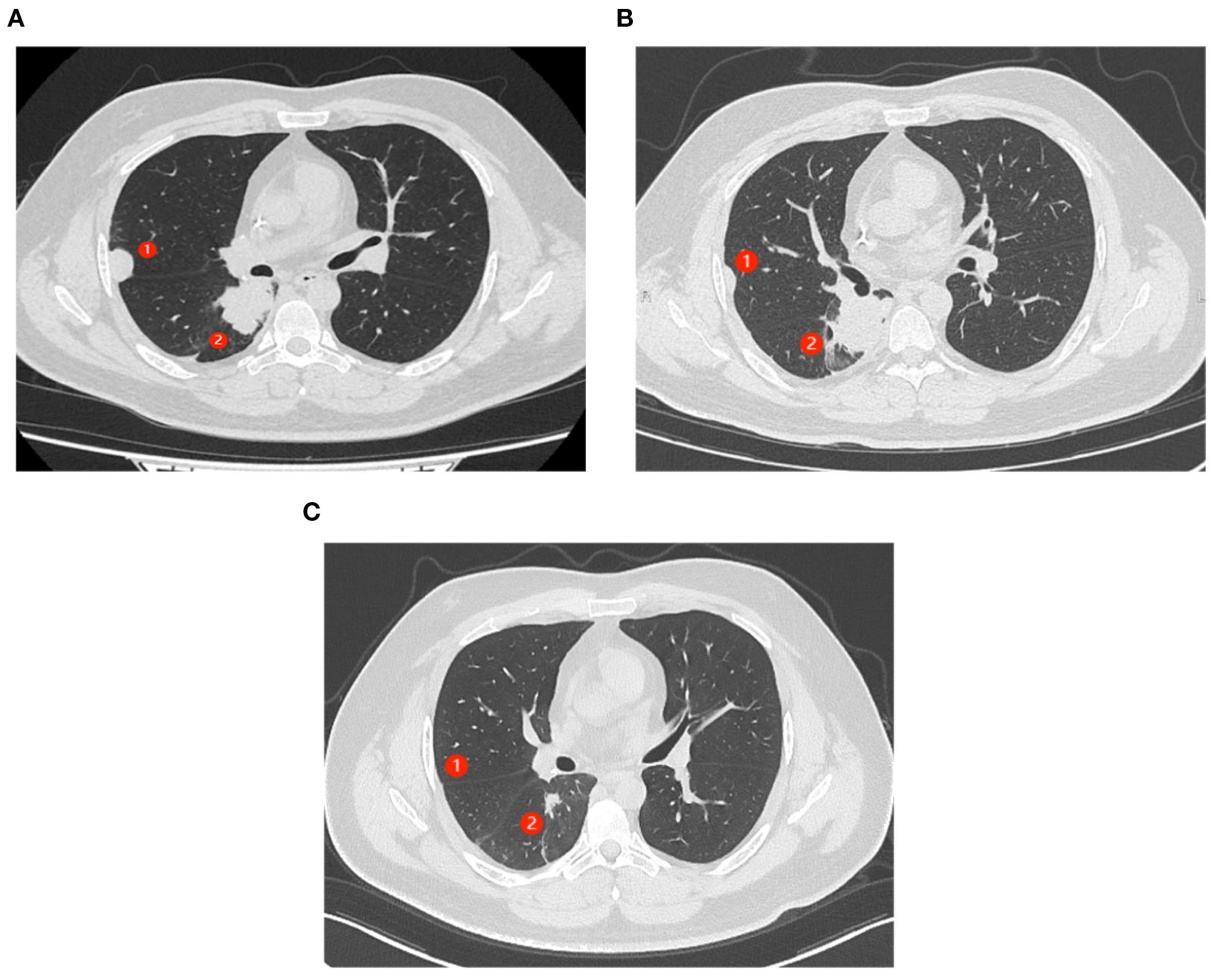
Abscopal response in a patient with nephroblastoma following radiofrequency ablation (RFA). ① represents the lesion near the chest wall that did not undergo RFA. ② represents the lesion in the hilar region where RFA had been performed. (A) CT scans of the patient’s chest show progressing lung lesions before the initiation of RFA. (B) CT scan shows the resolution of ① after 3 months of therapy (C). (C) CT scans (axial views) show complete response (CR) of ① and partial response (PR) of ② after 12 months of therapy.
